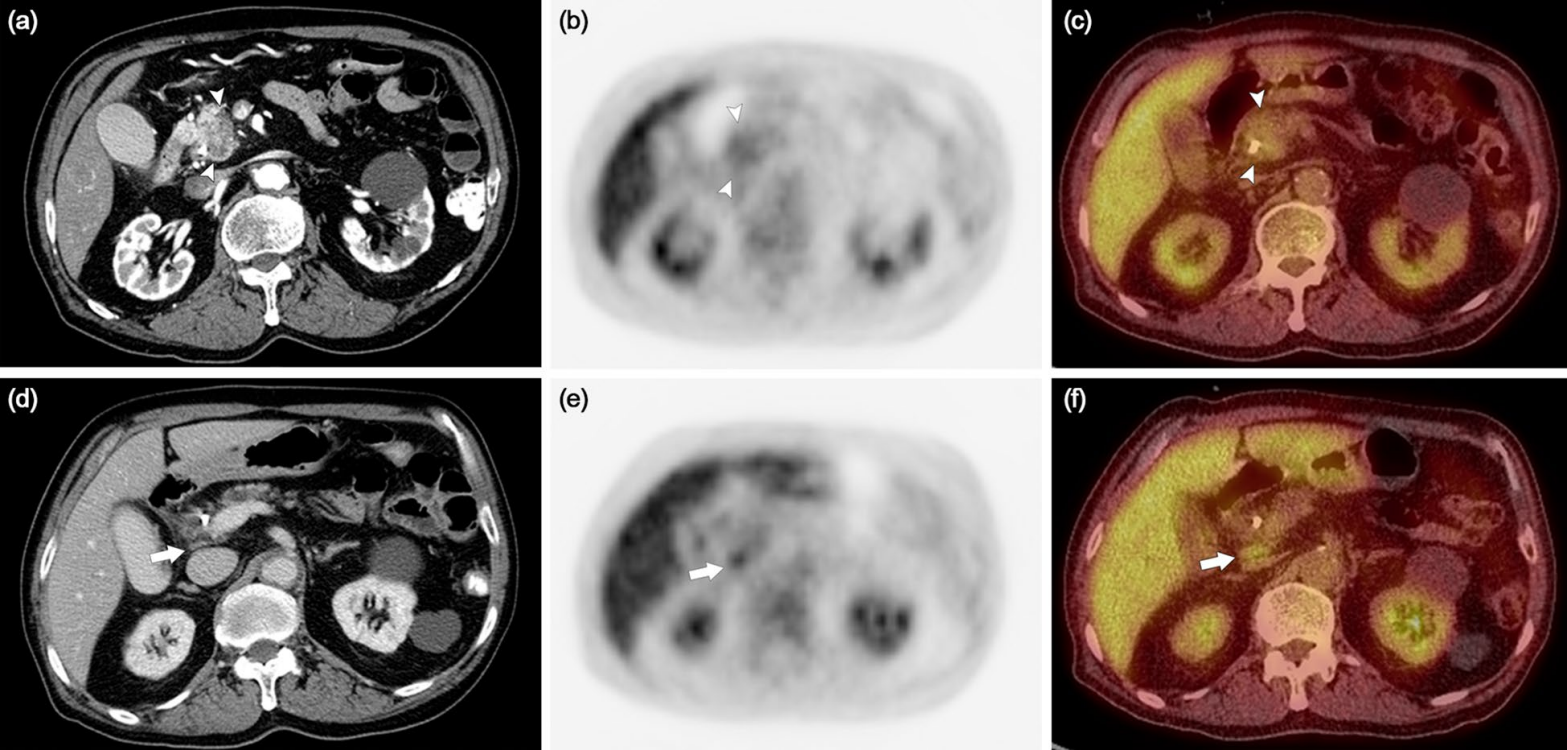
Contrast-enhanced dynamic computed tomography (CT) and ^18^F-fluoro-2-deoxyglucose-positron emission tomography (FDG-PET) scans of a 76-year old male patient diagnosed with pancreatic head cancer. A 2.7 cm sized hypoattenuating mass (arrowheads) is noted on the arterial phase of the CT (a), which shows significant FDG uptake on the FDG-PET (b) and FDG-PET/CT scans (c). A small lymph node (LN) was noted in the portocaval space (arrow) on the venous phase of the CT (d), with significant FDG uptake on the FDG-PET (e) and FDG-PET/CT scans (f). The patient was categorized as positive regional LN metastasis by the LN_OR_ definition, but negative regional LN metastasis by the LN_AND_ definition. After pylorus-preserving pancreaticoduodenectomy and regional LN dissection, regional LN metastasis was confirmed. Early local recurrence was identified on CT and FDG-PET scans performed 10.5 months after surgery. Patient expired 15.9 months after surgery.
